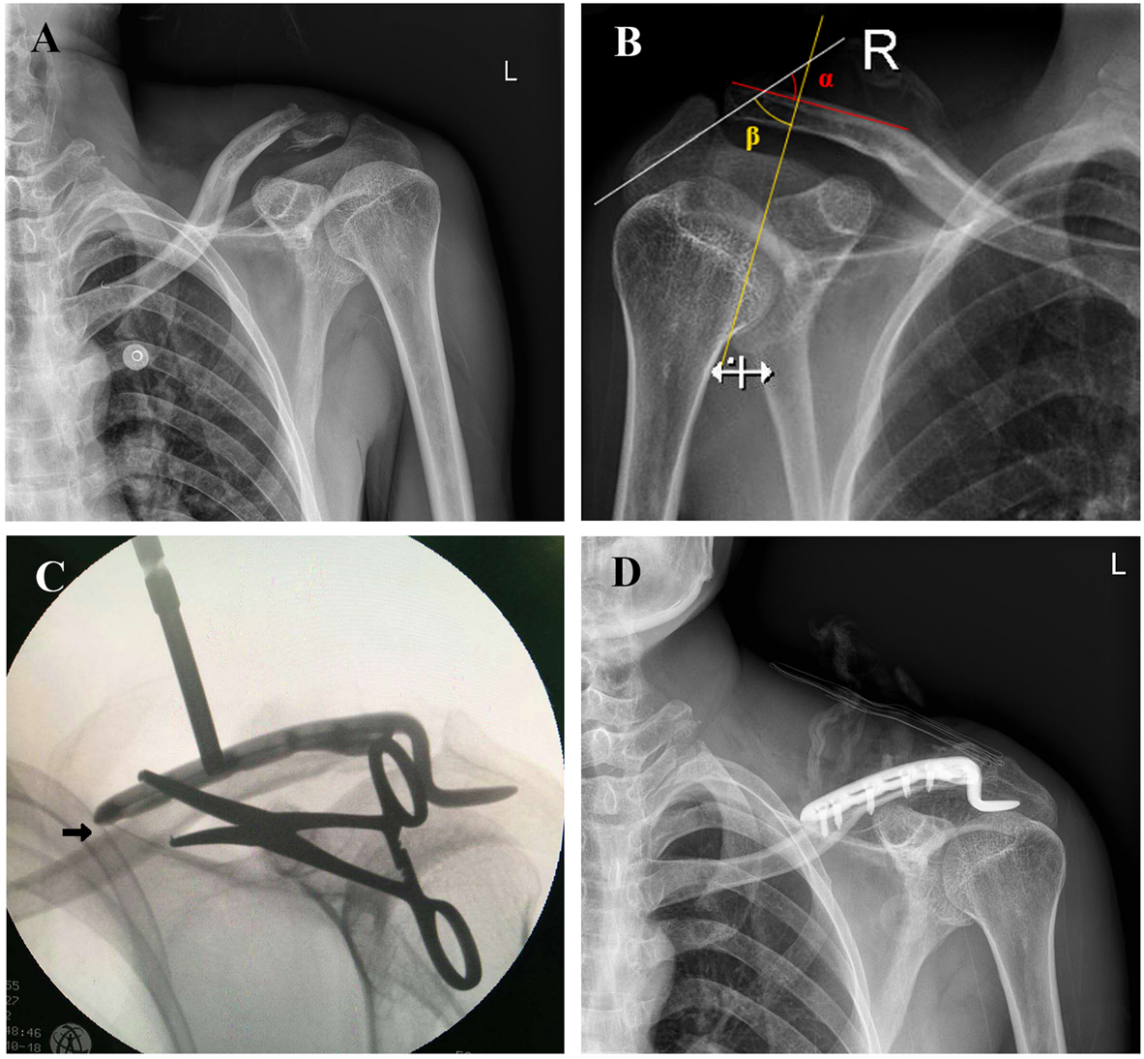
Case 1: A 30-year-old man with left distal clavicle fracture treated with clavicular hook plate. a Preoperative radiography indicated Neer type I fracture of the distal clavicle. b distal clavicle–acromion coronal angle (α) and acromion coronal angle (β) on X-ray films of uninjured side. c Intraoperative fluoroscopy showed the endpiece of plate uplifted (black arrow). d Postoperative X-ray demonstrated accepted fracture reduction
